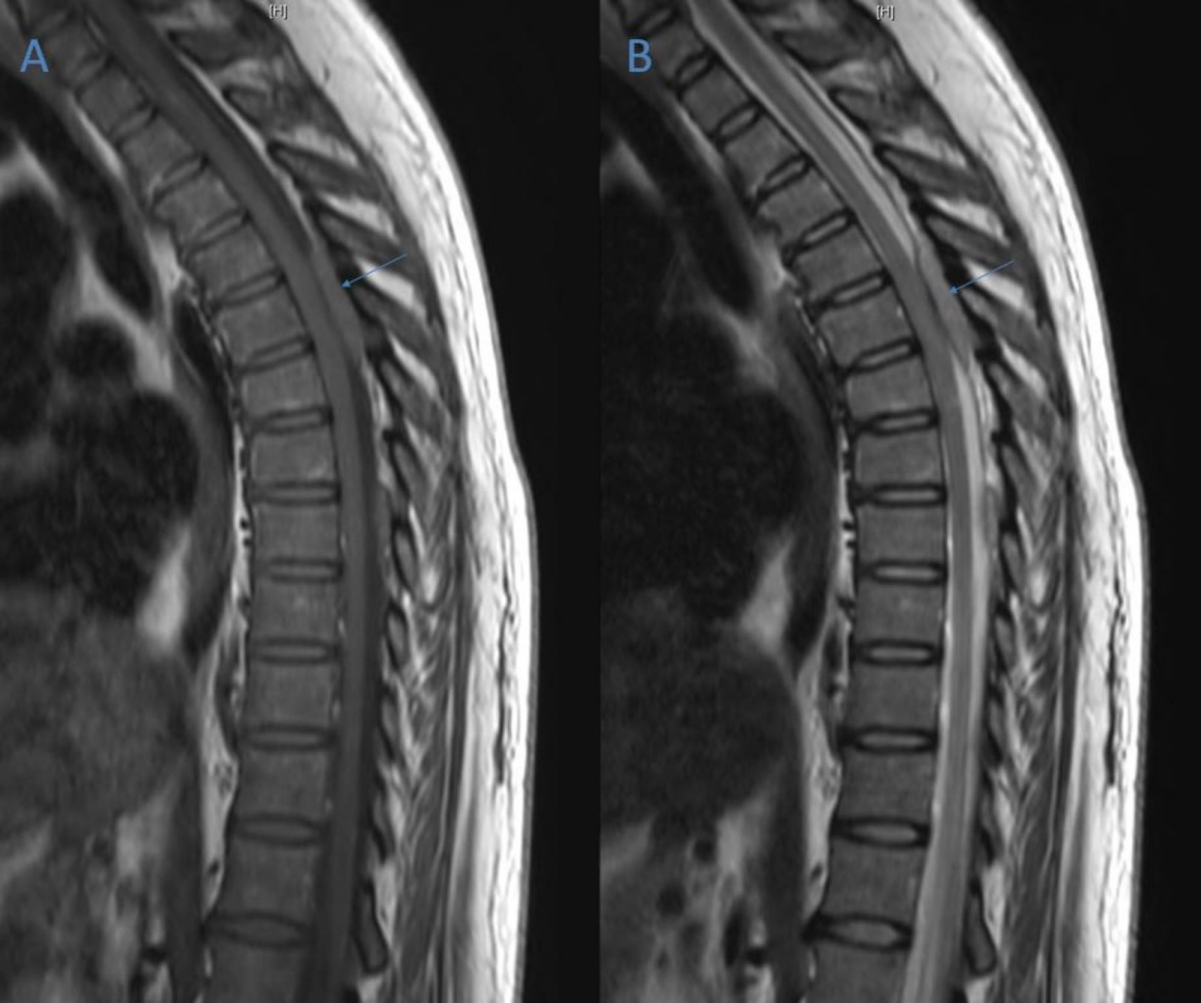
Sagittal Magnetic Resonance Imaging (MRI) from Day One of Admission. (A) Gadolinium-enhanced T1-weighted sagittal MRI showing posterior epidural lesion centered at T5 vertebral level (arrow). (B) Gadolinium-enhanced T2-weighted sagittal MRI showing posterior epidural lesion centered at T5 vertebral level (arrow).
